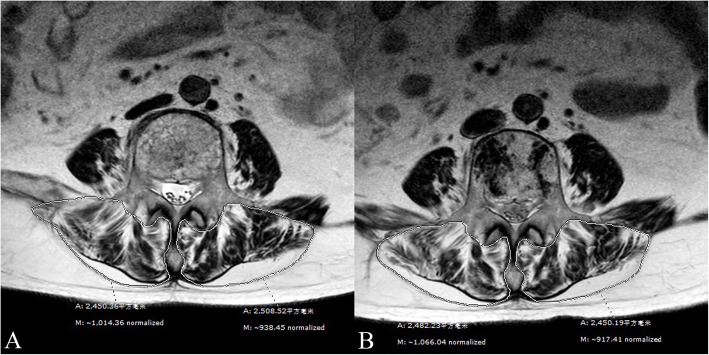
A 66-year-old woman with a OVCF at L4. A Preoperative axial T2-weighted image of L4 was measured. B Routine scan after 11 months reported no refracture occurrence. *A* areas, *M* mean grayscale
